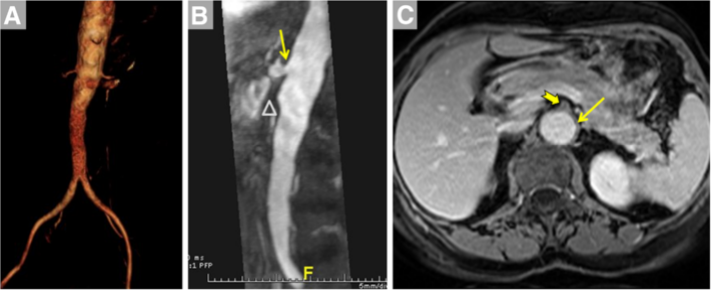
Images of the abdominal aorta in a patient with Takayasu arteritis are shown.A. Volume rendering viewed in the antero-posterior direction of a CE-MRA acquired using a coronal slab demonstrates marked irregularity of the infraceliac abdominal aorta. B. Maximum intensity projection of a CE-MRA obtained using a sagittal slab at a subsequent visit shows ostial disease of the celiac trunk (arrow) and absence of the superior mesenteric artery (SMA, open triangle). C. Post-contrast volumetric interpolated breathhold T1-weighted image shows thickening of the wall of the abdominal aorta (arrow) and obliteration of the SMA ostium (notched arrow).
